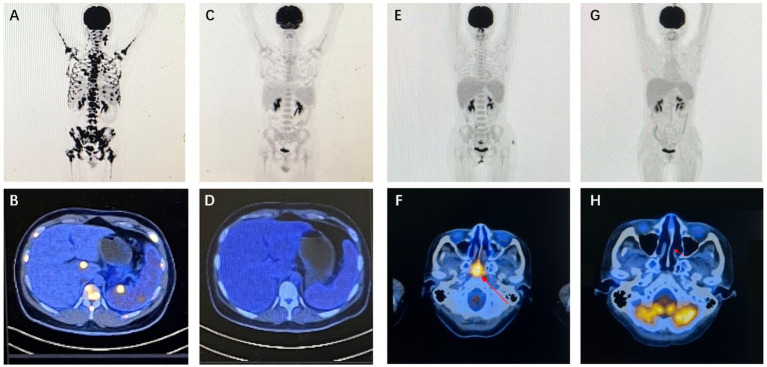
Imaging findings of representative patients. Case 20 PET/CT results: baseline PET/CT demonstrated systemic lymph node involvement, splenomegaly with focally increased metabolic activity, and concurrent intramedullary lesion infiltration (A,B). Following two cycles of P3-GemOx therapy, follow-up PET/CT confirmed complete resolution of all FDG-avid lesions, achieving complete response (CR) (C,D). Case 22 PET/CT results (study obtained after initial chemoradiotherapy): baseline PET/CT revealed soft tissue thickening with intensely increased metabolism in the posterosuperior wall and bilateral walls of the nasopharynx, suggestive of neoplastic lesions (E,F). After two cycles of P3-GemOx therapy, follow-up PET/CT documented complete resolution of all FDG-avid lesions, confirming complete response (CR) (G,H).
